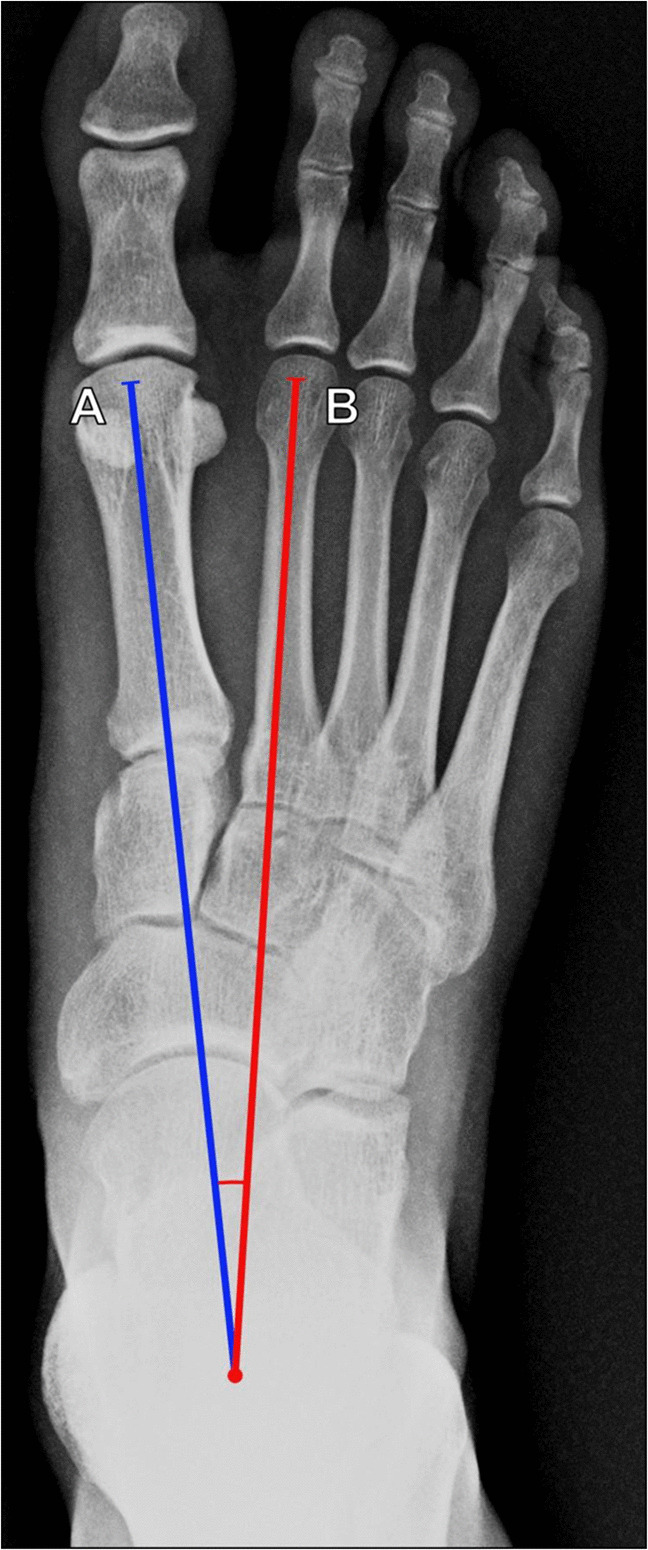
Measurement of first–second intermetatarsal angle on DP radiograph of the foot. The angle is formed between the long axis of the first metatarsal (A, blue line) and the long axis of the second metatarsal (B, red line). An angle ≥ 9° is associated with hallux valgus
